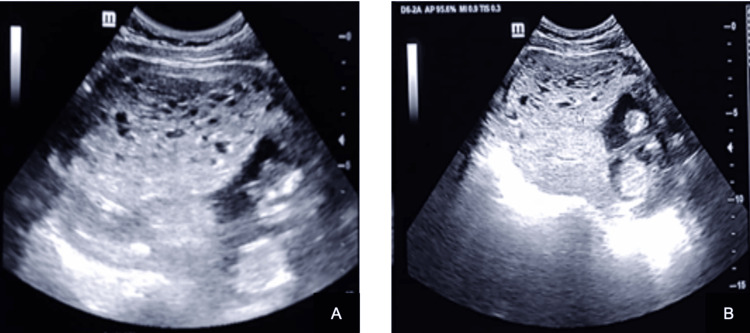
Obstetric ultrasound findings Ultrasound images demonstrating a multicystic placental mass with a “snowstorm” appearance (A) coexisting with a live fetus (B), findings suggestive of molar pregnancy.
